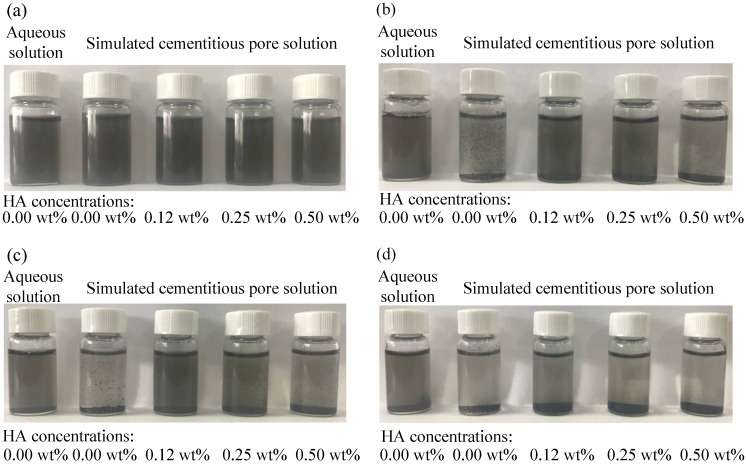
MWCNT suspensions containing different concentrations of humic acid (HA) in cementitious pore solutions and aqueous solution at different times: (a) 0 h; (b) 3 h; (c) 6 h; (d) 18 h after ultrasonication.
